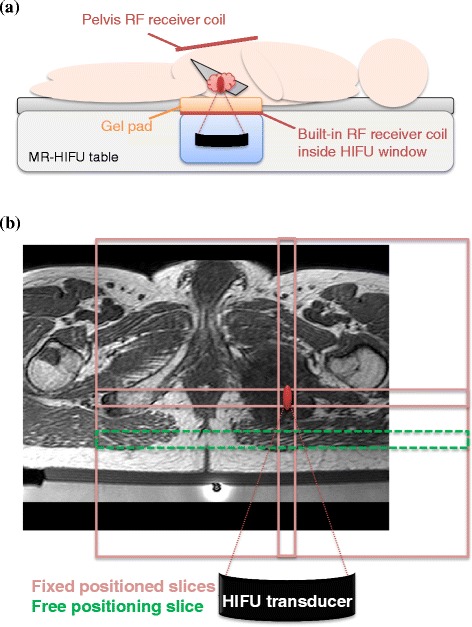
Slice positioning of the MRT scans. An example of an MR-HIFU setup for a treatment in the pelvis is shown in (a). An example of the MRT scan slice positioning is shown in (b), on a T1-weighted planning scan of an osteolytic lesion in the pubic bone (treatment 10). Three slices (light-red) were fixed with the centers to the location of the HIFU focus; one slice could be freely placed by the user and was placed in the near-field area of the HIFU beams (green, dashed).
